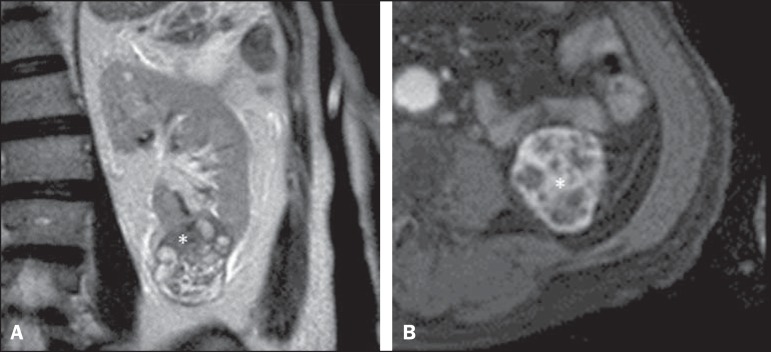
Coronal T2-weighted (A) and axial post-contrast T1-weighted
